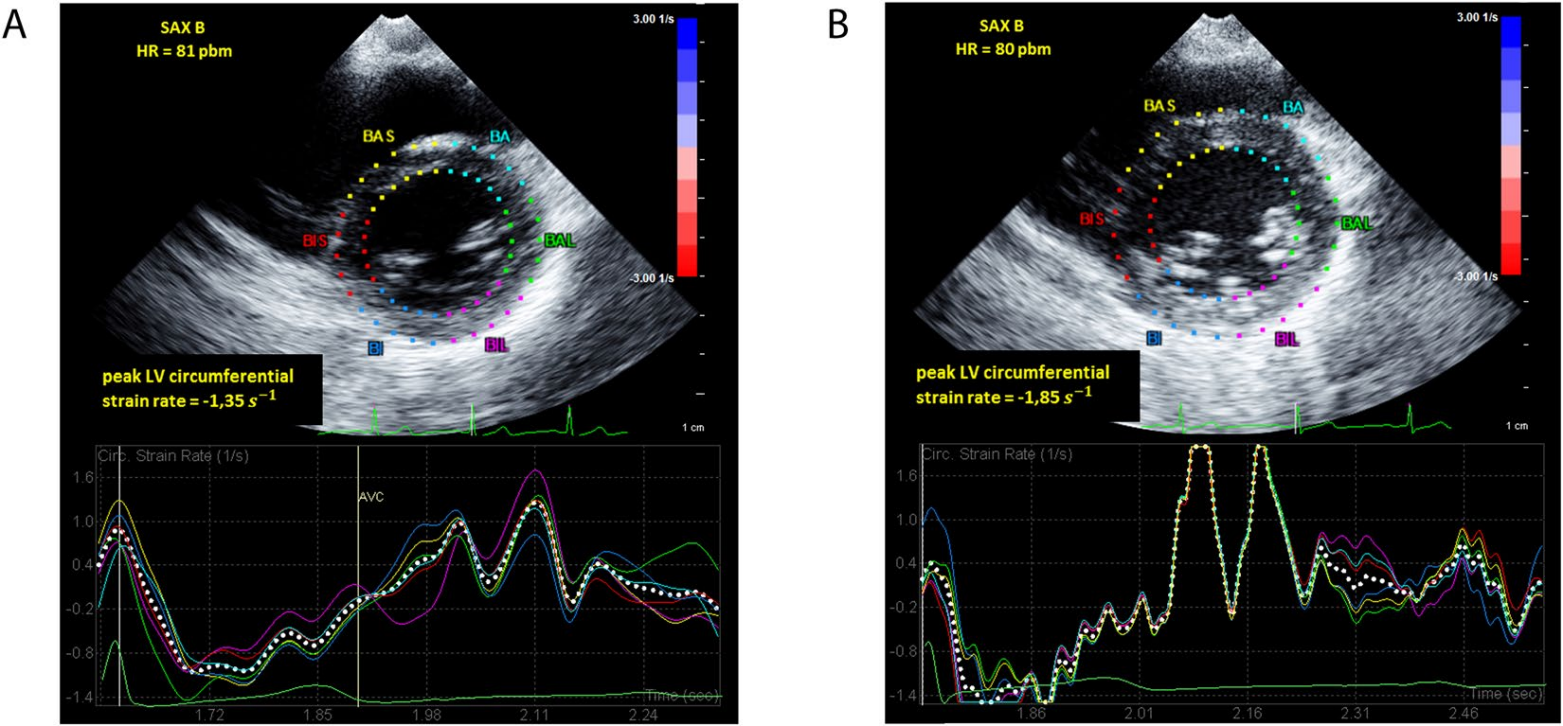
Echocardiographic short axis view derived circumferential strain rate at rest in a patient with active CD (left) and a healthy control (right). *Note the significantly decreased peak LV circumferential strain rate in the child with IBD*.
